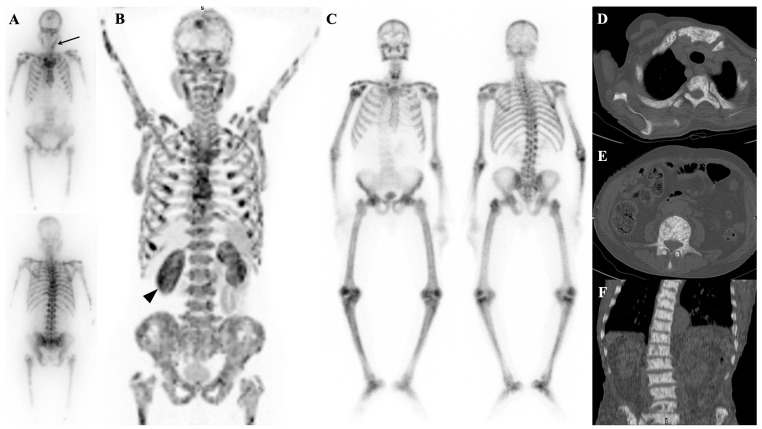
Double superscan, also known as super-superscan. A case of a prostate cancer patient with coexistent metastatic superscan and secondary hyperparathyroidism (PTH = 294 pg/mL, calcium = 7.6 mg/dL, Phosphorus = 2.6 mg/dL, and Creatinine = 1 mg/dL). Note the mandibular uptake (arrow) and absence of kidneys in the first post-treatment scan of ^177^Lu-PSMA-617 (A). Also, note the visualization of the kidneys in the pre-treatment ^68^Ga-PSMA-11 scan ((B), arrowhead). The underlying mechanism for discordant kidney uptake in the ^177^Lu-PSMA-617 and ^68^Ga-PSMA-11 is unknown. Another case of an elderly patient with a history of prostate cancer presents with progressive anemia and generalized weakness. A recent bone scan reveals atypical findings compared to the typical metastatic superscan observed in prostate cancer. Notable features include a prominent mandible and significant appendicular uptake (C). Upon examining fused images, widespread bone sclerosis is evident (D–F). The differential diagnoses considered are myelofibrosis/myelosclerosis or hyperparathyroidism superimposed on a metastatic superscan.
